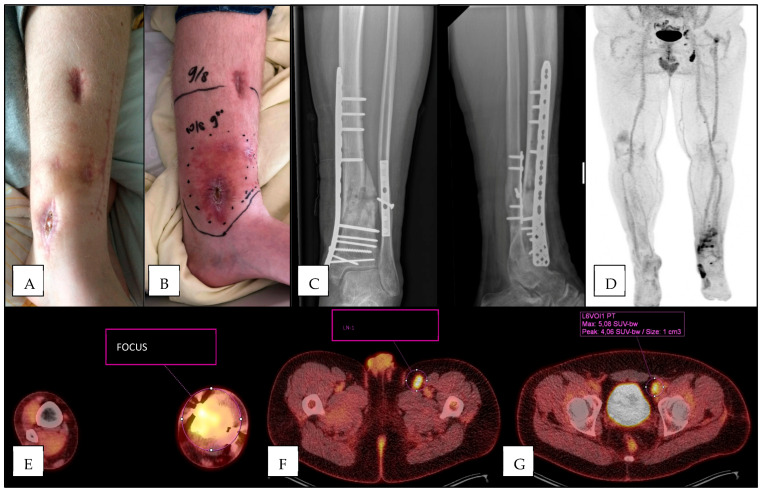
Patient presenting 17 months after distal tibia fracture, and three months after non-union treatment with autologous cancellous bone graft. Initially, there were no clinical confirmatory signs (A), though progressive pain and erythema developed in the following weeks (B) suggestive for FRI. Plain radiograph showed non-union after plate- and screw osteosynthesis (C). [18F]FDG-PET/CT was suggestive for FRI with increased uptake at the fracture site (D,E), and showed inguinal (F) and iliac (G) lymph nodes with increased uptake. Intra-operatively obtained deep tissue cultures were positive for *Staphylococcus epidermidis*.
